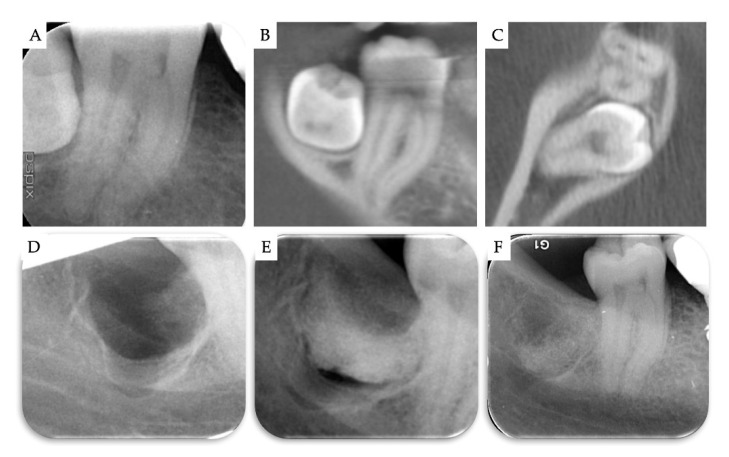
Case no 4. (A–C) Periapical X-ray and CBCT images at T0. Periapical X-ray (D) immediately after the removal of M3, and (E) immediately after grafting the bone defect with β-TCP/CS. (F) 1 year post-operatively.
